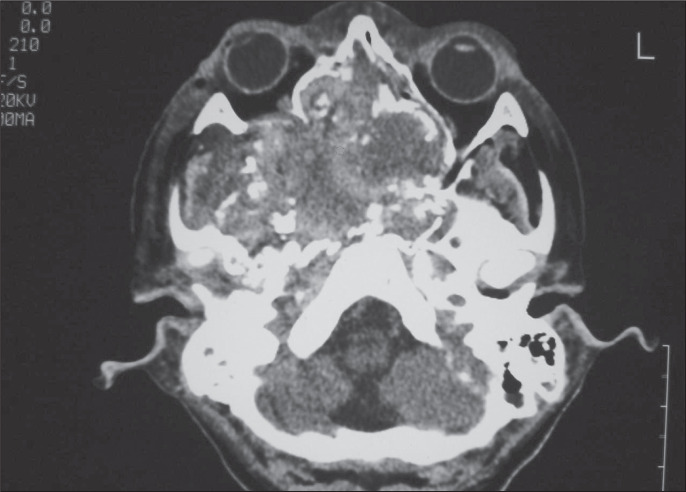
Computed tomography scan of a twelve-year-old patient, showing lesion of soft tissue density invading maxillary fossa, eroding the skull and middle and nasal fossa.
